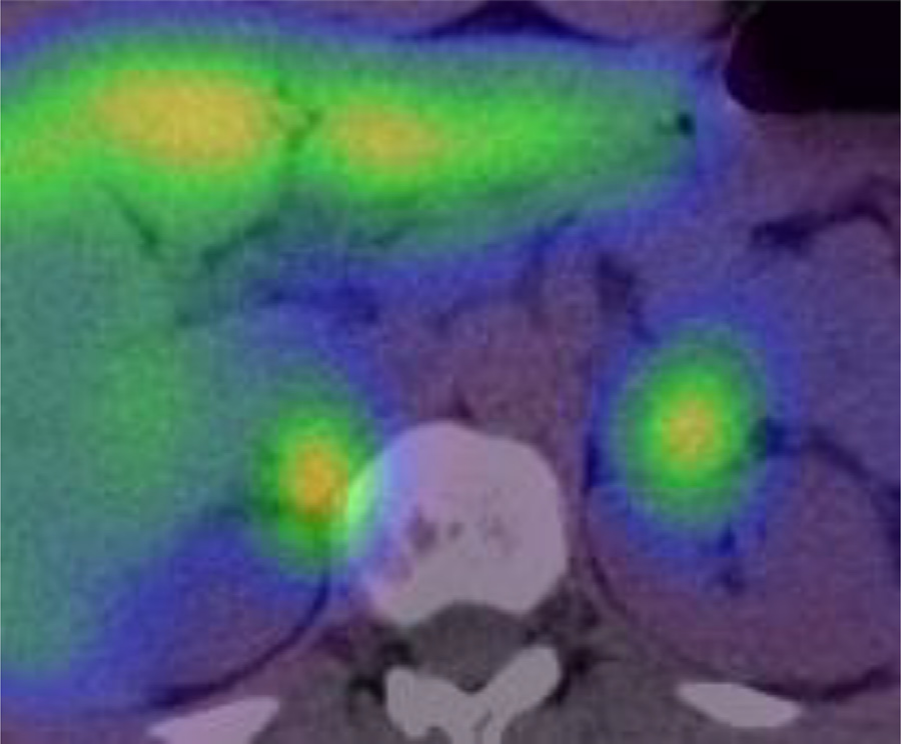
^131^I-MIBG scintigraphy. Both adrenal lesions exhibit apparent MIBG accumulations, consistent with bilateral pheochromocytomas.
